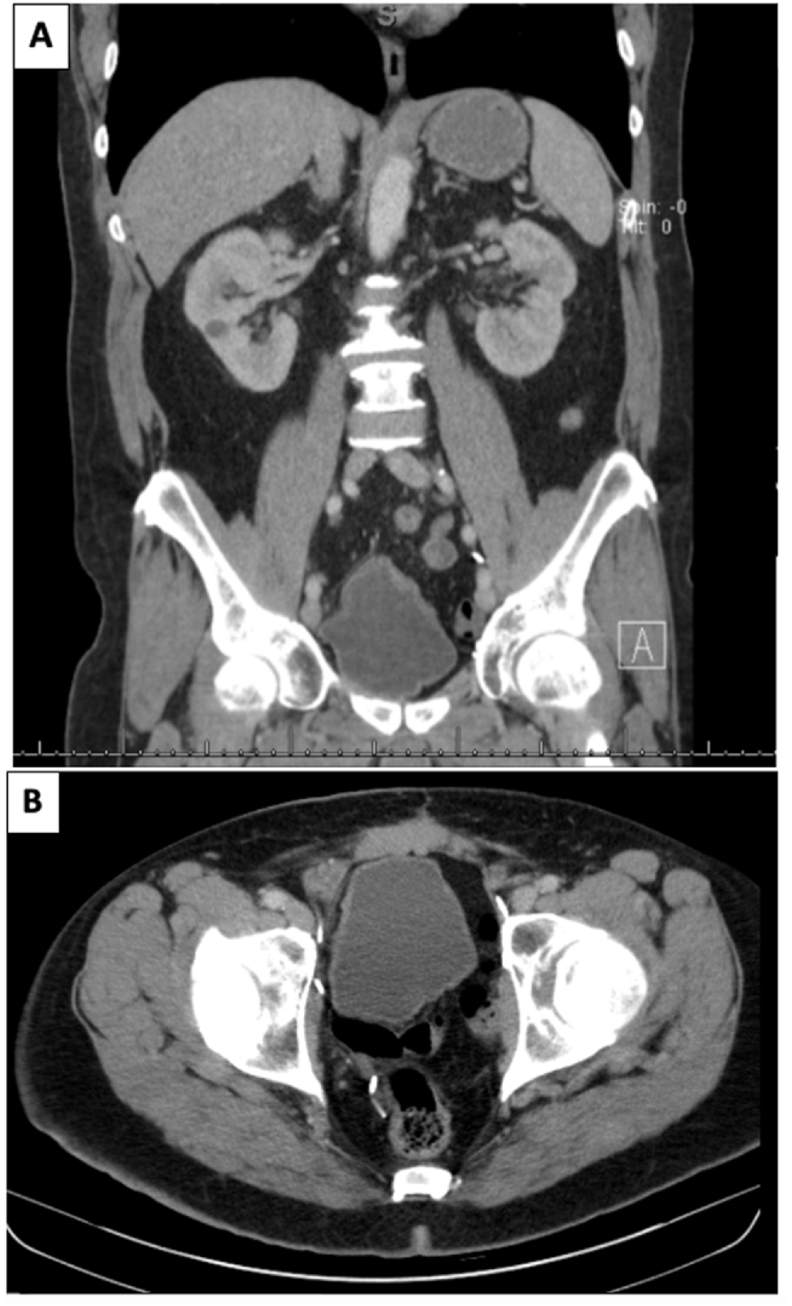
8-Months Post-Operative CT Abdomen and Pelvis with Contrast Demonstrating Intact Neobladder with no Evidence of Lymphadenopathy or Metastatic Disease. A) Coronal. B) Axial.
